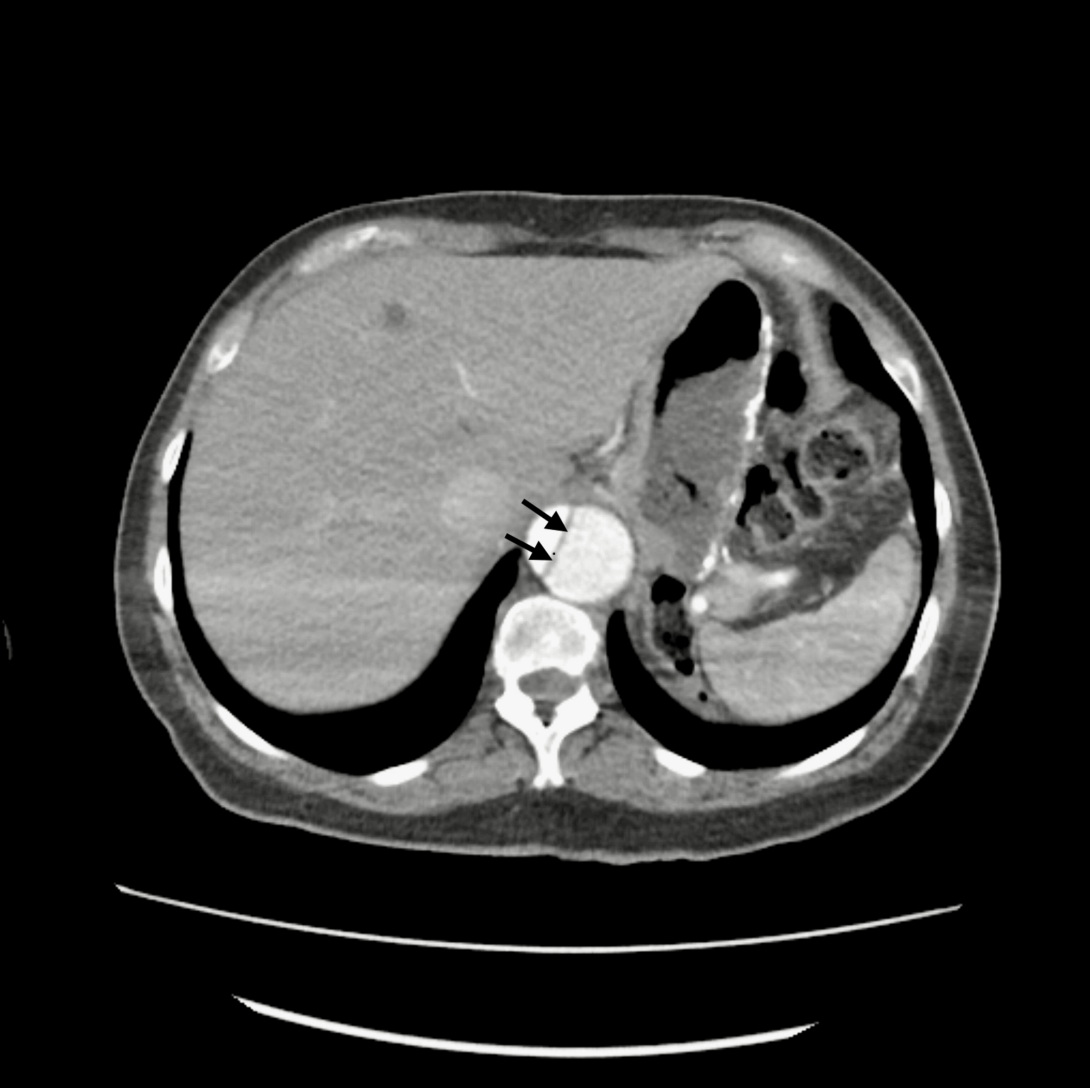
CTA scan axial images of the upper abdomen with a repaired DeBakey type 1 dissection. Arrows indicate the false lumen of the dissection CTA: computed tomography angiography
